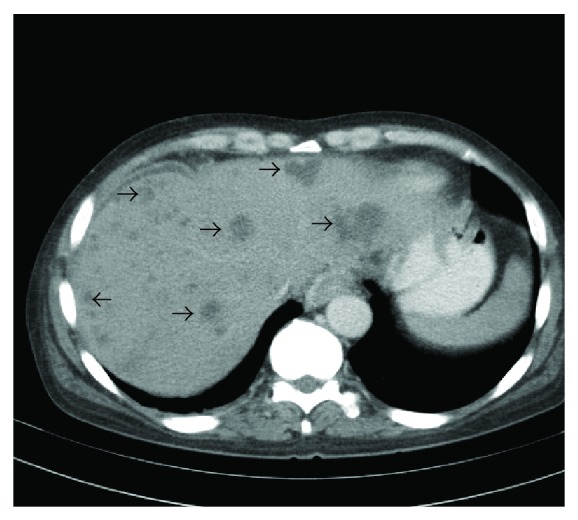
CT scan of the abdomen highly suspicious for multiple hepatic metastases.
